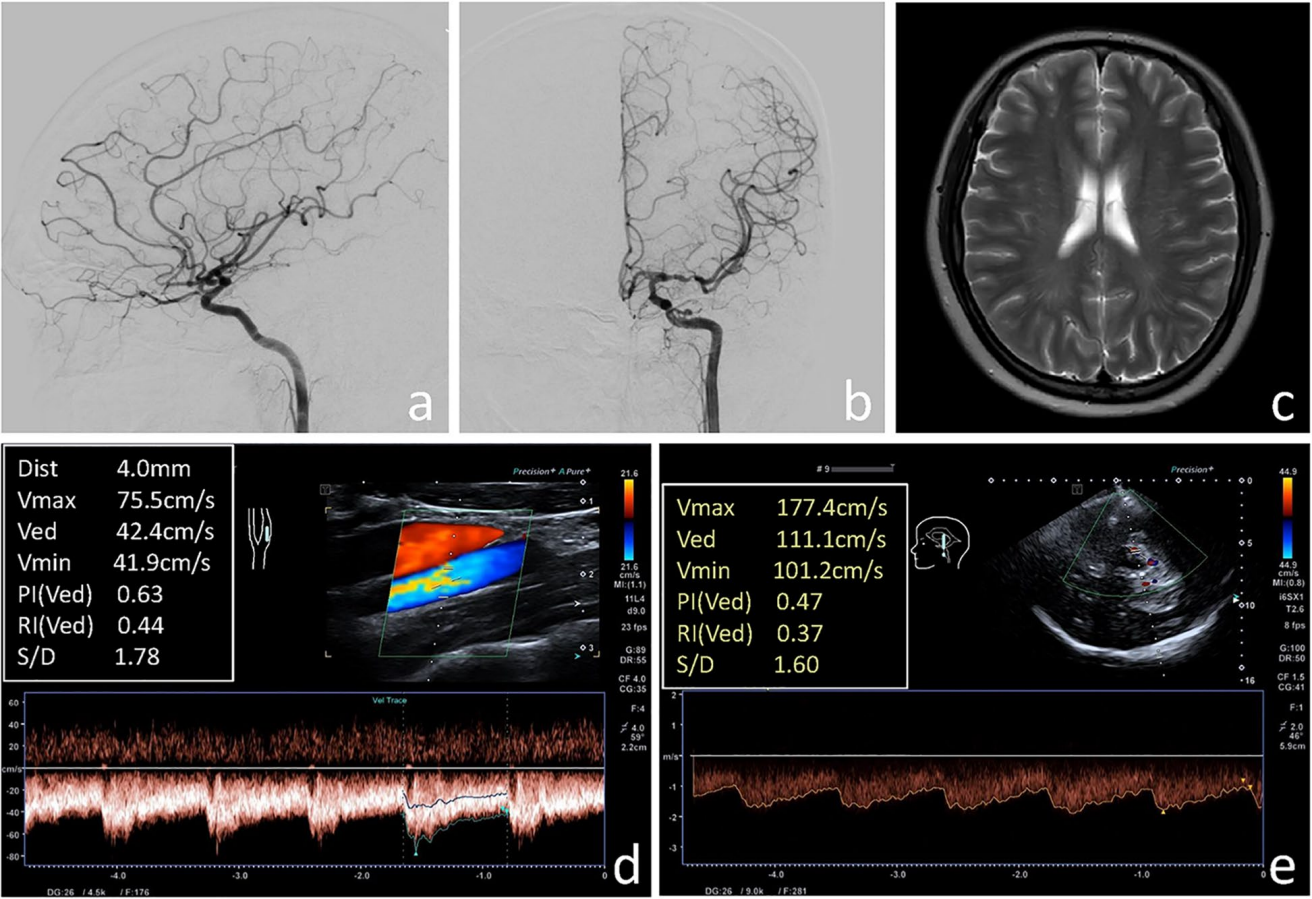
a, b Digital subtraction angiography confirmed moyamoya disease in the left hemisphere. c Brain magnetic resonance imaging showed no obvious abnormalities in the left hemisphere. d Ultrasound examination of the left internal carotid artery. e Transcranial color-coded duplex sonography of the left posterior cerebral artery
